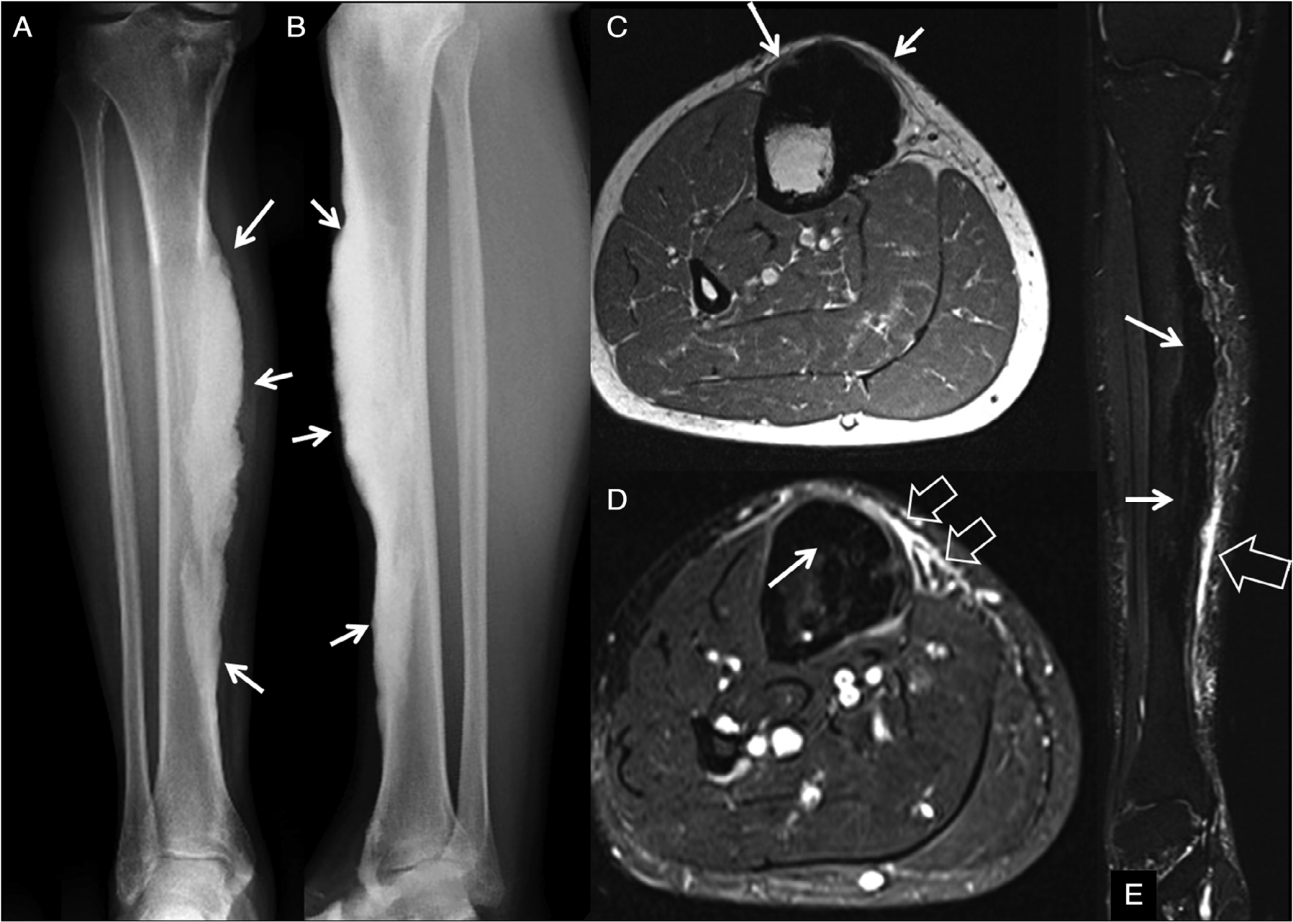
“Shin splints” in melorheostosis. A 46‐year‐old male (Melo‐2) patient with a follow‐up of 8 years after initial diagnosis. Anteroposterior (*A*) and oblique (*B*) plain X‐rays of the lower limbs, showing the dripping candle‐wax lesion located in the tibial diaphysis (arrows). The axial T1‐weighted (*C*), fat‐suppressed proton density‐weighted (*D*), and coronal short tau inversion recovery (*E*) MR images show the dripping candle‐wax lesion returning low signal on all pulse sequences (arrows). MRI shows in addition parosteal edema on the anteromedial aspect of the tibia similar to shin splints (open arrows).
